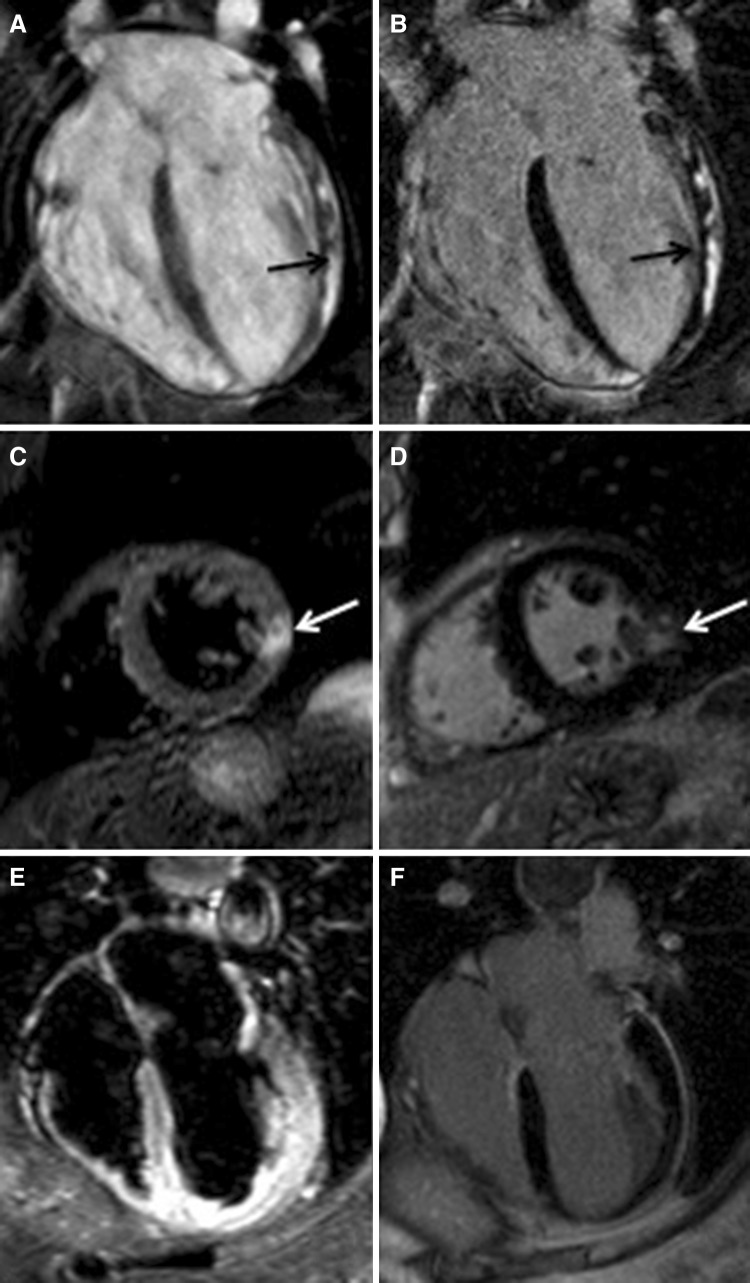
Acute coronary syndromes with normal angiogram. Early gadolinium enhancement sequences showing extensive epicardial enhancement (a, *black arrow*) and corresponding late gadolinium (LGE) on the post-contrast sequences (b, *black arrow*) in a patient with acute myocarditis. T2-weighted images showing focal discrete oedema in the mid-cavity inferolateral wall (c, *white arrow*) with corresponding LGE on post-contrast sequences (d, *white arrow*) in a patient with embolic myocardial infarction in the mid left circumflex territory. T2-weighted sequences showing acute myocardial oedema in the apical segments (e) with no evidence of late gadolinium enhancement (LGE) on the post-contrast sequences (f) in a patient with Tako-Tsubo cardiomyopathy
